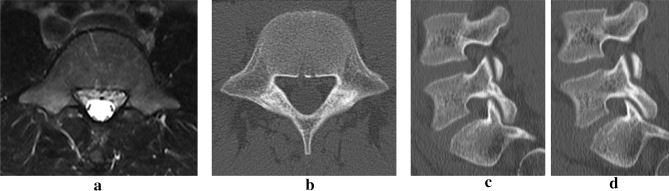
Nineteen weeks after the first examination, the intensity change on MRI disappeared (a). The fracture lines disappeared on the axial CT slice (b) and there was no obvious worsening of the resorption in the sagittal view (c, d).
